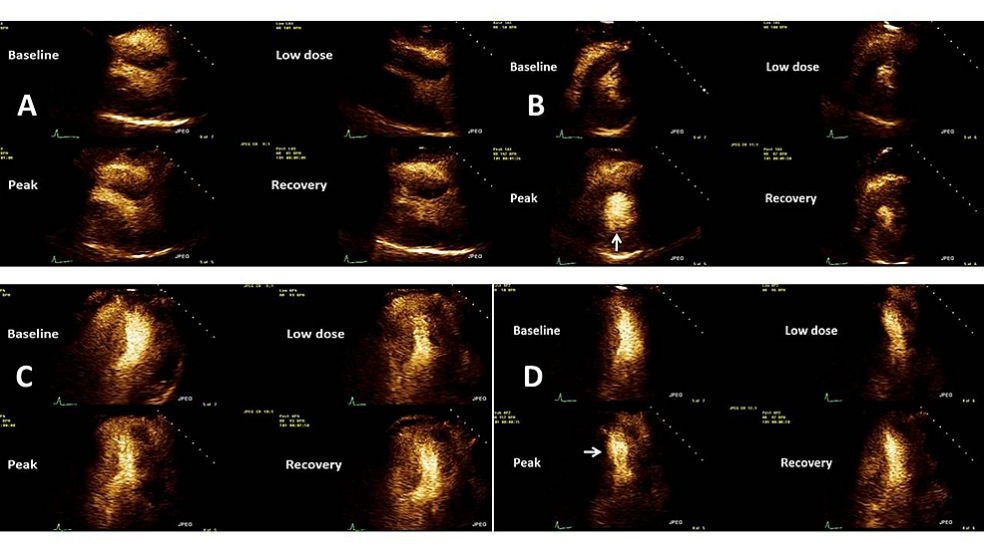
Stress echocardiogram from clinical case 1 (A) Standard parasternal long-axis views of the left ventricle showing baseline, low dose, and peak dose followed by recovery images. (B) Standard short-axis views of the left ventricle showing the same test phases as in the previous. In this set, please note that the arrow demonstrates a lack of contractility of the inferior wall in this view. Inferior wall contractility improves in the recovery images. (C) Standard four-chamber apical views of the left ventricle showing the same test phases as in the previous. (D) Standard two-chamber apical views of the left ventricle showing the same test phases as in the previous. Once again, in this set, please note that the arrow demonstrates a lack of contractility of the inferior wall. Once again, inferior wall contractility does improve in the recovery images
